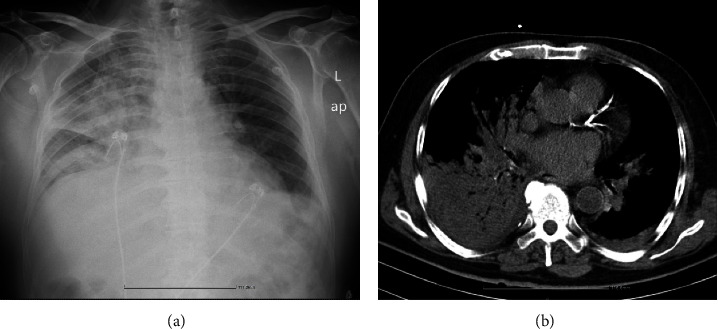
(a) Chest X-ray (bedside) and (b) chest CT scan on admission.
